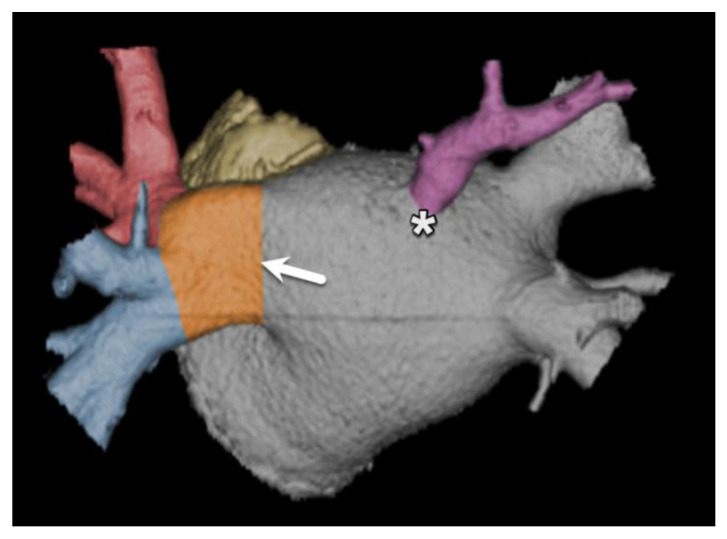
Volume-rendered CT image of the left atrium and pulmonary veins with anatomical variations. Both a common drainage (common trunk) of the left pulmonary veins (arrow) and a contralateral accessory pulmonary vein (asterisk) can be seen.
